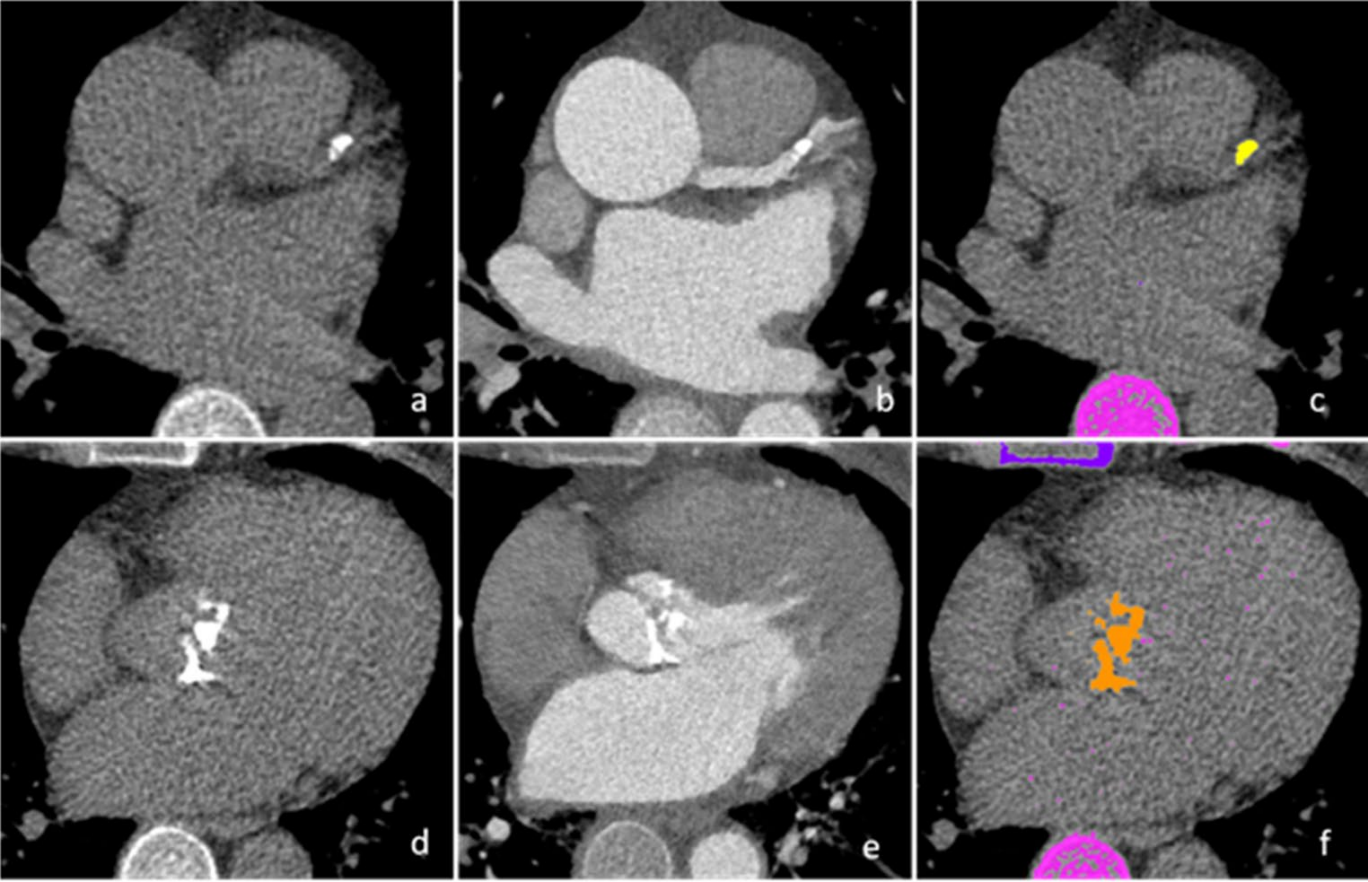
Graphic visualisation of measurements of Agatston-Score values on pre-TAVI CT imaging. On non-enhanced cardiac CT imaging (a, d), calcified lesions were identified and matched with the contrast-enhanced images (b, e). Afterwards, the calcified lesions were colour-coded and the Agatston-Score values were determined using imaging postprocessing software (SnygoVia, Siemens). In images a-c, evaluation of the LAD is visualised while in images e-f evaluation of the aortic valve is displayed
